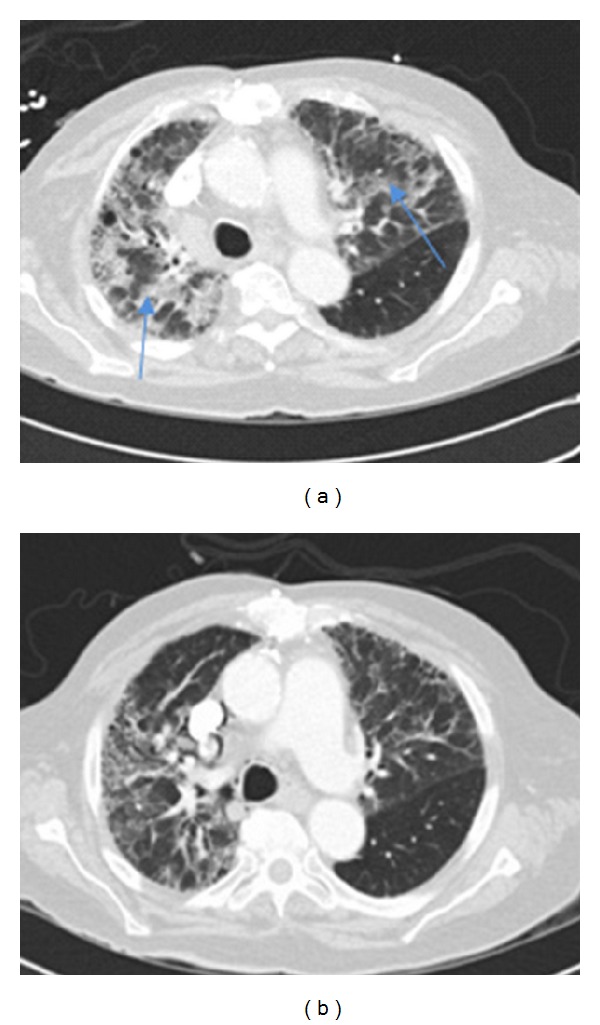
Computed tomography (CT) of the chest at the initial presentation of patient on the fifth day following rituximab treatment (a) and 20 days after initiation of steroid treatment (b). Arrows show bilateral pulmonary patchy infiltrates.
